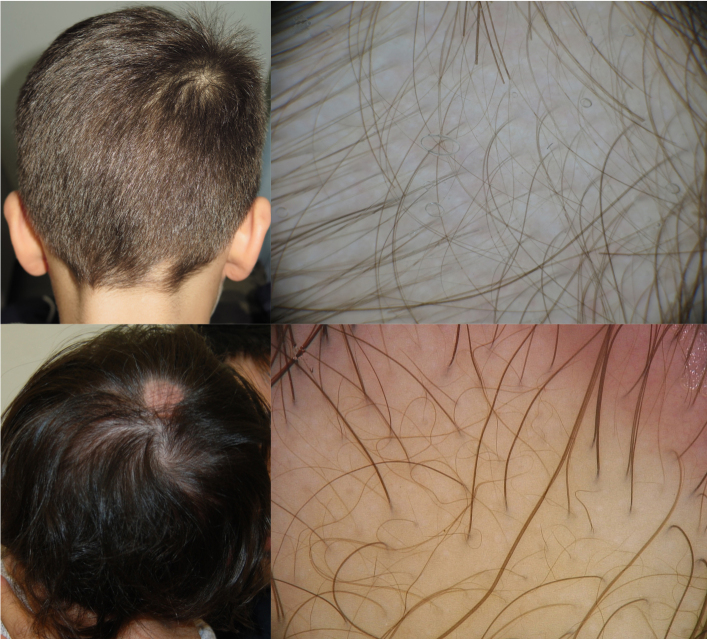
Two cases of our series: (A) occipital CTA; (B) vertex CTA. With trichoscopy, vellus hairs are evident (C, D), but also empty follicles (D). CTA = congenital triangular alopecia.
